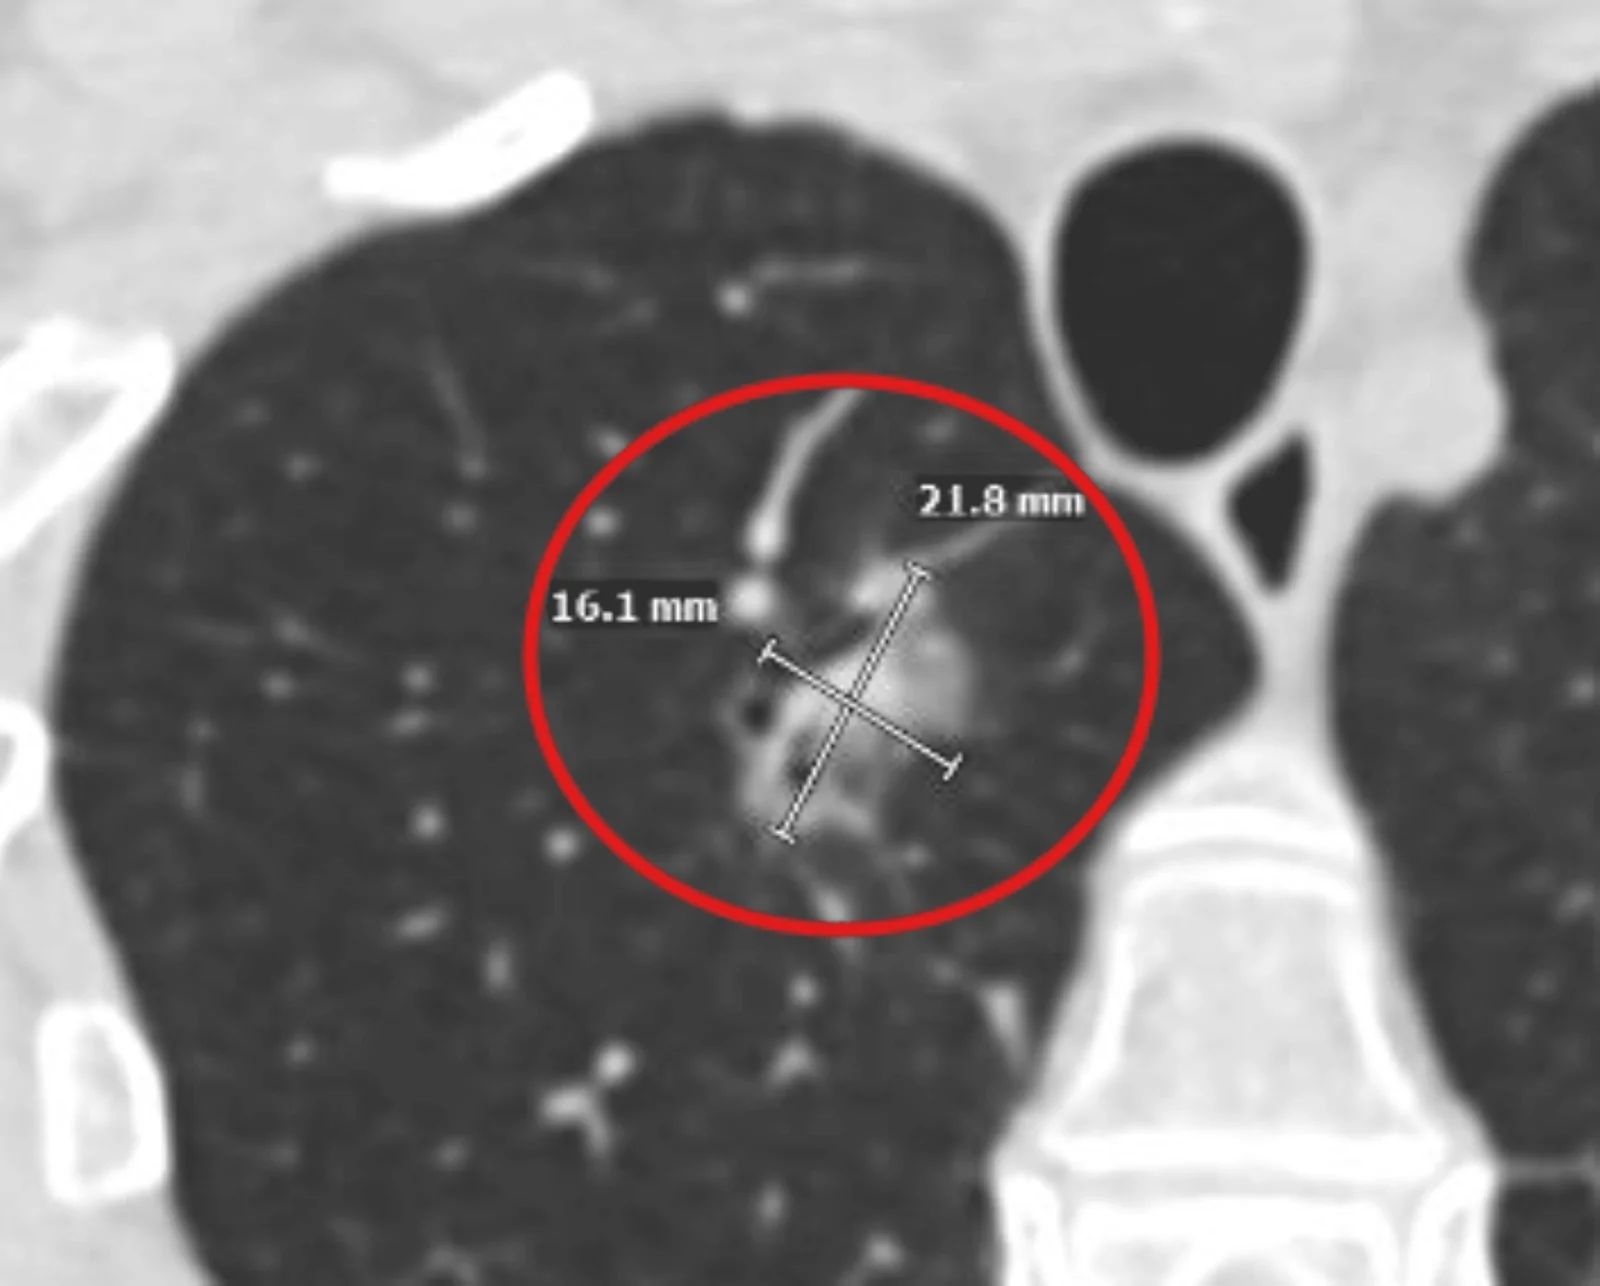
Selected axial non-contrast chest CT image obtained approximately nine years after the initial diagnosis, demonstrating progressive enlargement of the right upper lobe ground-glass nodule, with an increasing solid component compared with prior surveillance imaging.
